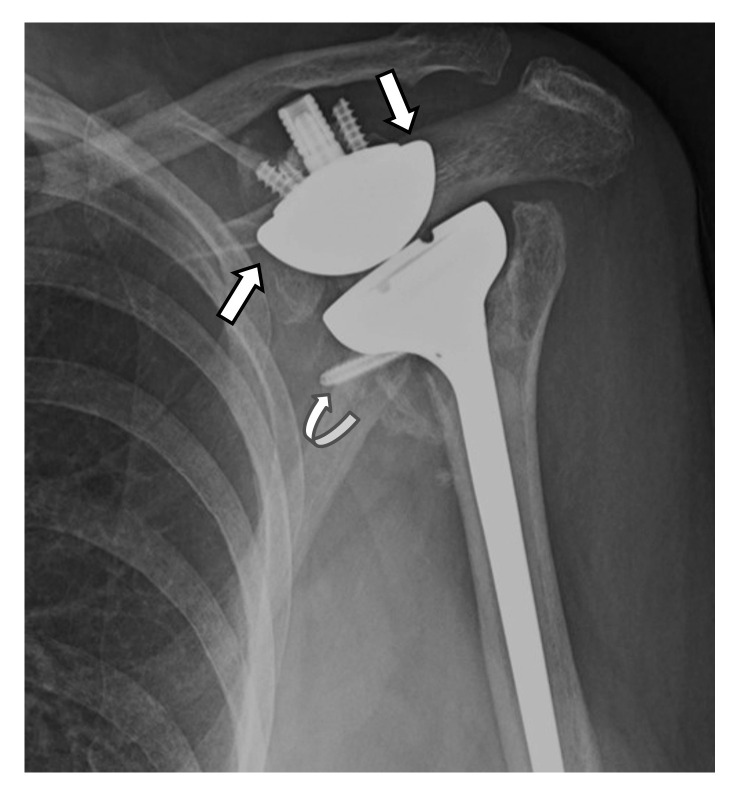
Frontal radiograph in a patient with RTSA shows fracture/break of a screw (curved arrow) as well as superior migration and rotation of the baseplate/metaglene (arrows), now dissociated from the glenoid.
